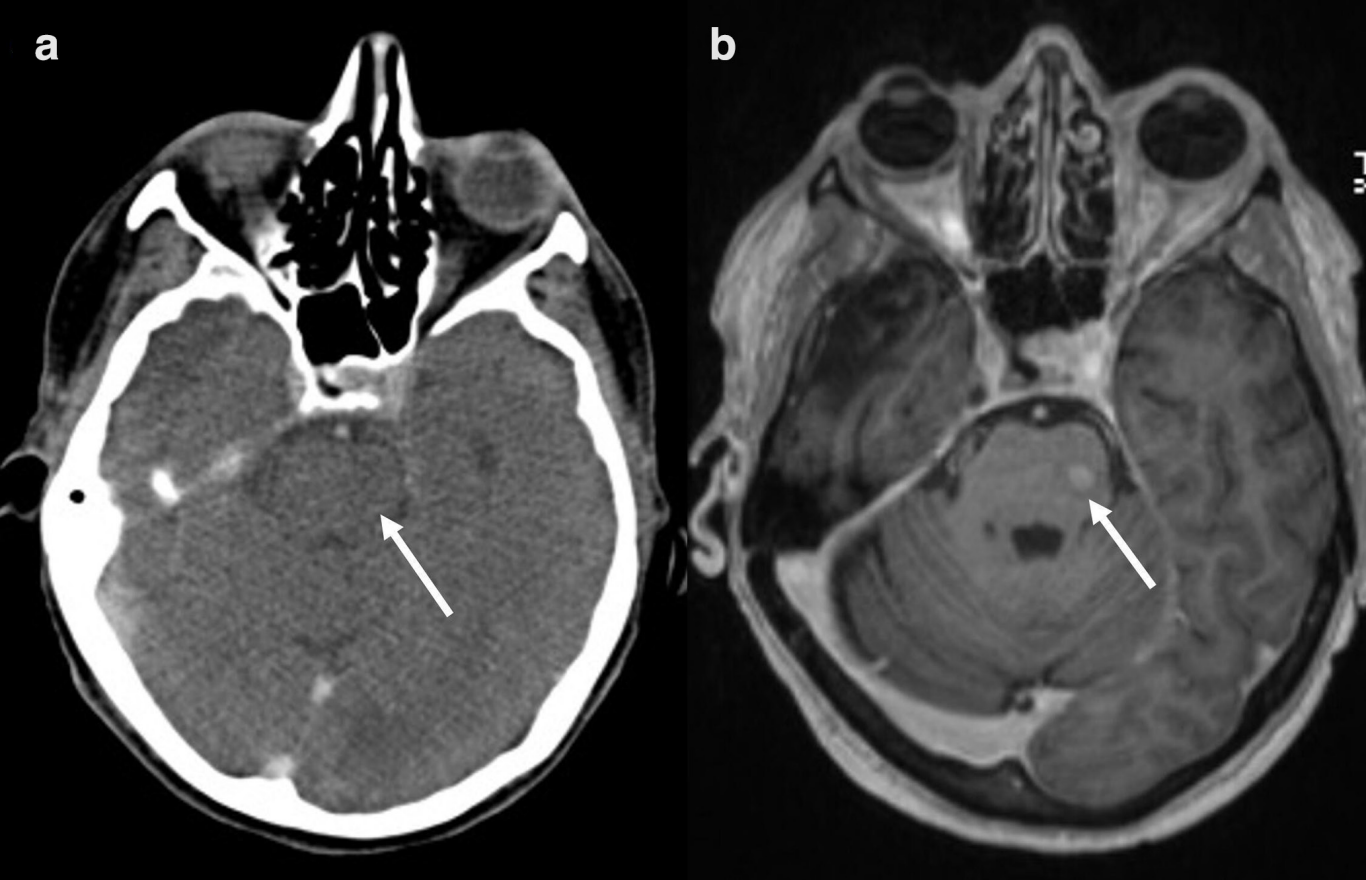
Axial a) contrast-enhanced CT and b) MRI of the neurocranium in a patient with newly diagnosed adenocarcinoma who was neurologically asymptomatic at the time of imaging. The CT scan showed no abnormalities, whereas there was a focal, increased contrast uptake in the left brainstem highly suggestive of a cerebral metastasis. The lesion was proven to be malignant in the follow-up examination by the pattern of growth.
